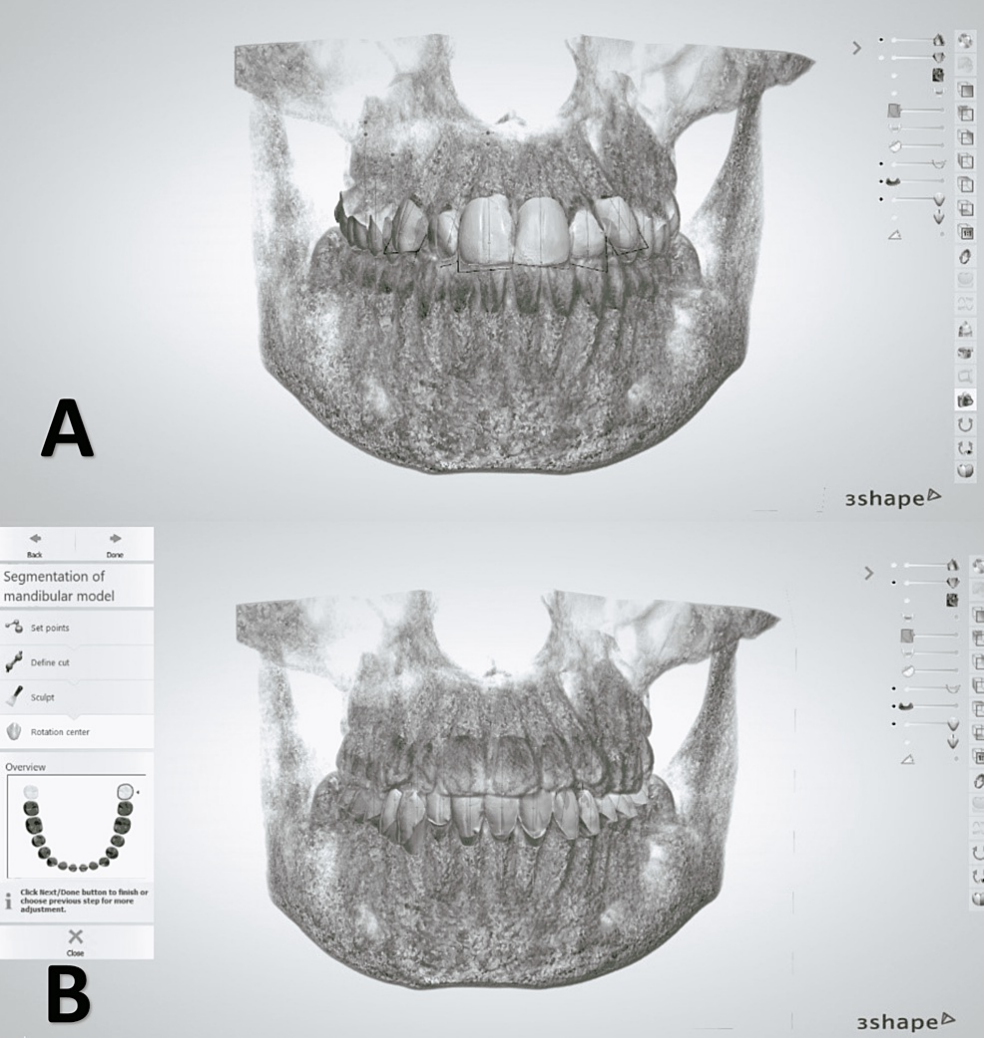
With the help of the anatomical information derived from the CBCT data, the long axis for each tooth is determined (A), and the center of rotation is located (B)
